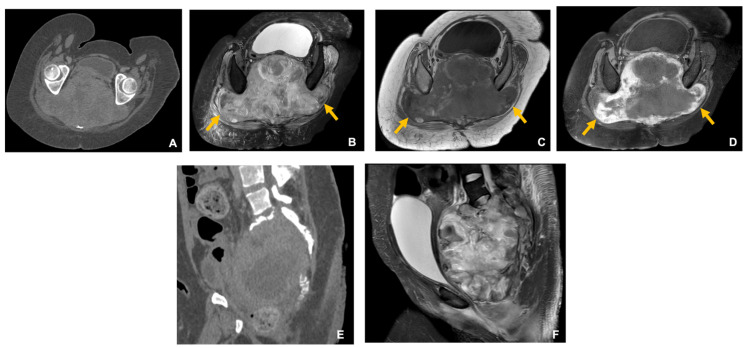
Giant cell tumor of bone in a 33-year-old woman. Axial (A) and sagittal (E) CT images show a very large osteolytic sacral lesion with cortical destruction. MRI sequences—axial (B) and sagittal (F) T2-weighted fat-suppressed, axial T1-weighted (C), and axial post-contrast fat-suppressed T1-weighted (D)—demonstrate intralesional T1 hyperintensity consistent with hemorrhage, low-to-intermediate T2 signal compatible with hemosiderin deposition, and heterogeneous enhancement. The lesion obliterates the expected pathways of the sciatic nerves (arrows).
